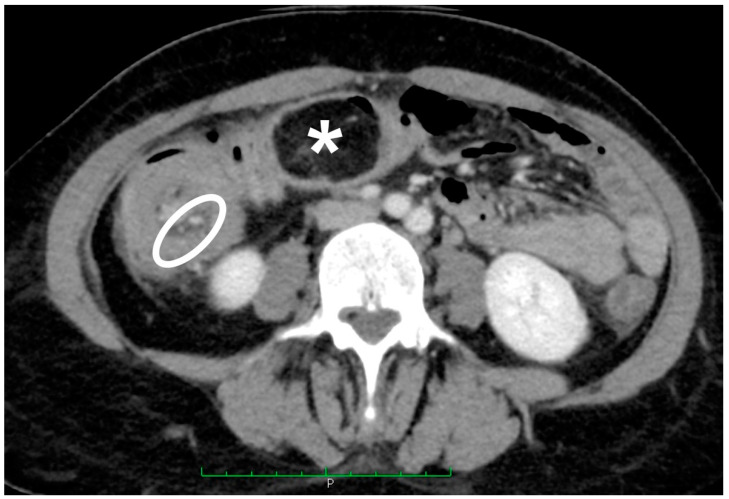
Axial CT scan of the abdomen in portal/venous phase. Circumferential thickening in ileocolic intussusception caused by the lipoma; note the mesenteric fat and vessels (white oval) and the terminal ileum associated with the intussuscipiens (asterisk).
